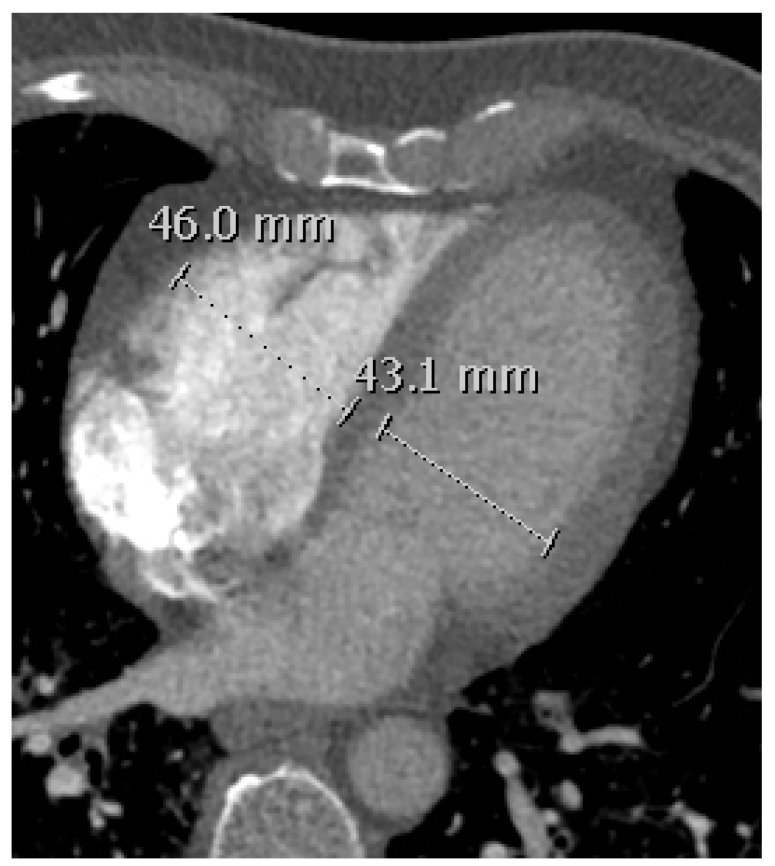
Measurement of right and left ventricle diameter using the axial method on a transverse CT angiography.
